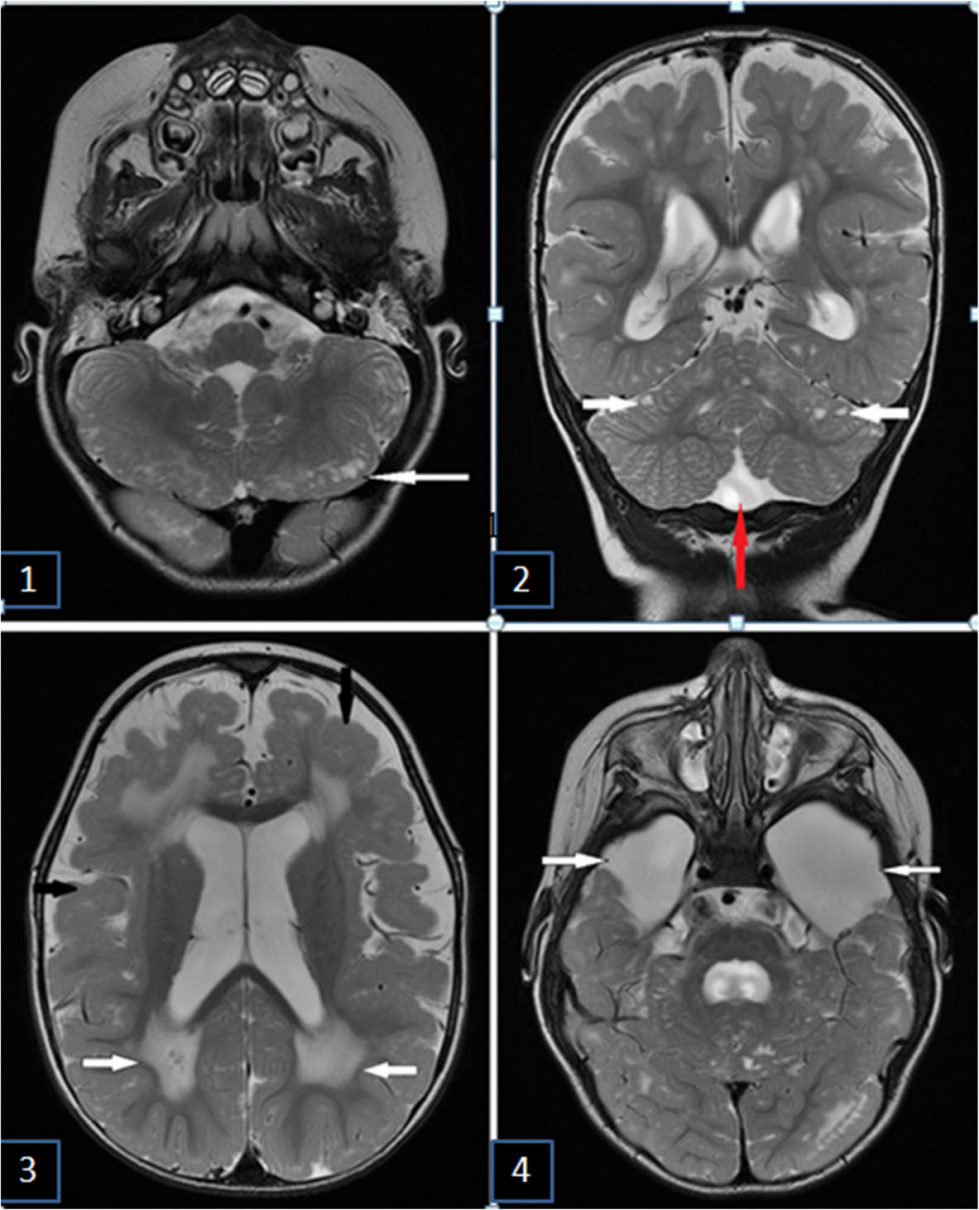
Brain MRI of the 6-year-old boy with dystrophy-dystroglycanopathy. 1 - Multiple subcortical cerebellar cysts (white arrow). Axial T2 weighted image. 2 - Hypoplasia of caudal parts of the cerebellum worm (red arrow) and subcortical cerebellar cysts (white arrows). Frontal T2 weighted image. 3 - Pachygyria-polymicrogyria of frontotemporal lobes (type “cobblestone pavement”) (black arrows) and demyelination of white matter (white arrows). Axial T2 weighted image. 4 - Hypoplasia of the temporal lobes (white arrows). Axial T2 weighted image
